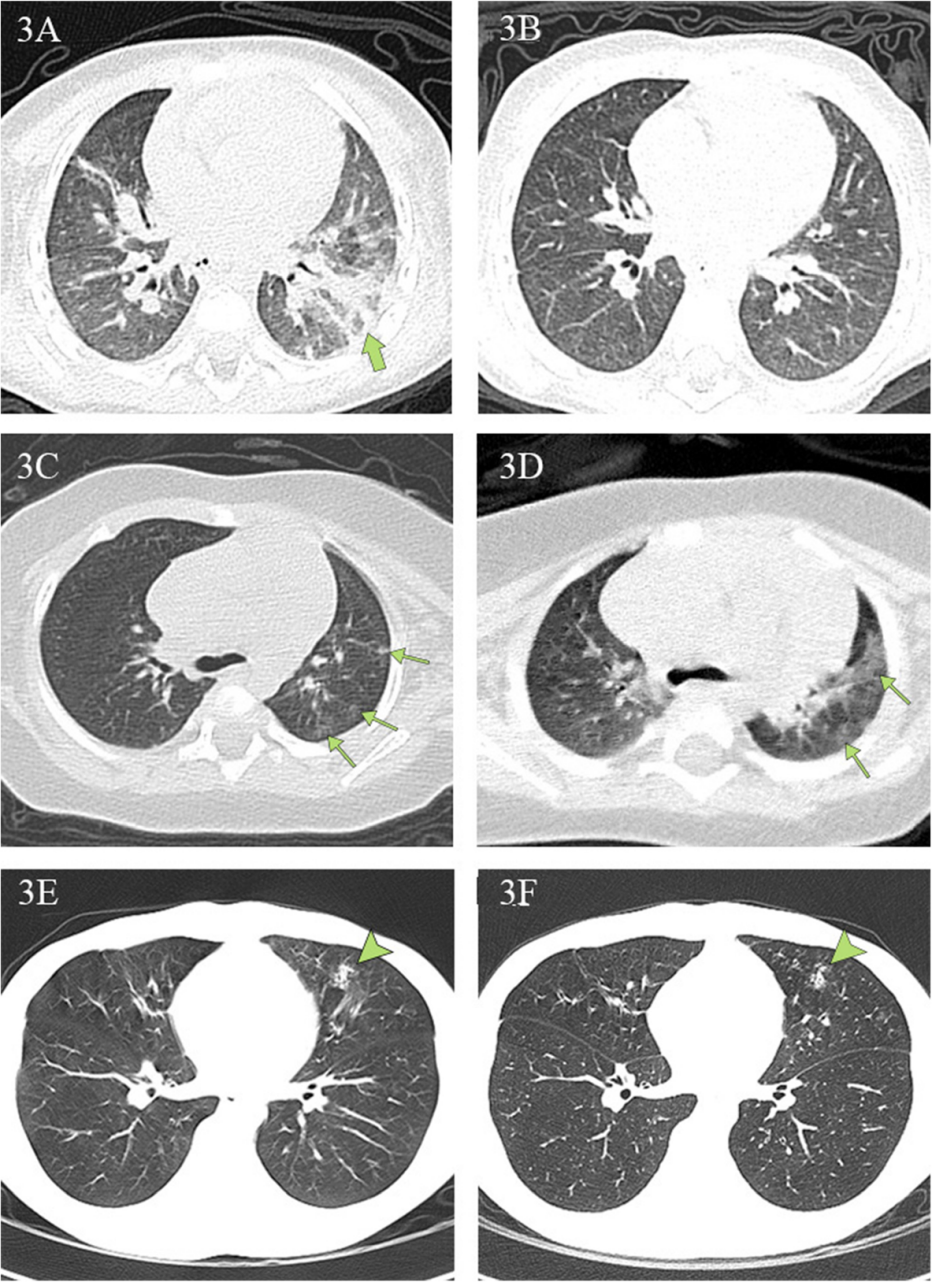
Chest CT findings at initial presentation and at discharge. 3A, 3B Chest CT scans obtained from a 1-year-old boy, presenting with fever and diarrhea, at arrival (3A) and after (3B) treatment. The first CT scan shows a large, patchy shadow in the left inferior lobe (green arrow). The second CT scan shows no lesions. The patient was hospitalized for 17 days prior to discharge. 3C, 3D Chest CT scans from a 4-month-old girl, who presented with a fever and a cough at arrival. The first CT scan reveals multiple ground-glass opacities under the pleura in the left superior lobe (green arrows). The second CT scan reveals that the range of original lesions was enlarged and extended to the center. The girl was hospitalized for 13 days and subsequently discharged. 3E, 3F Chest CT scans from a 14-year-old boy, presenting with rhinorrhea and a cough, at arrival and discharge. The first CT scan reveals a patchy shadow in the left middle lobe (arrowhead). There were no obvious changes in the areas of pulmonary consolidation on the second CT scan. The boy was hospitalized for 11 days and then discharged
